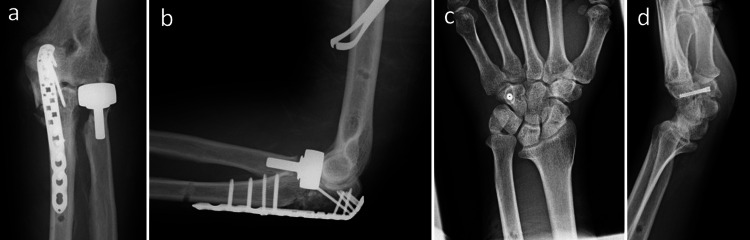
Plain X-ray image after the second surgery. (a) Anteroposterior and (b) lateral plain radiographs of the left elbow. Olecranon fracture was fixed with a locking plate. The fractured radial head was replaced with an artificial radial head. (c) Anteroposterior and (d) lateral plain radiographs of the left wrist. The non-displaced hook of the hamate fracture was fixed with a headless compression screw. The distal radioulnar joint remains dislocated after the second surgery.
